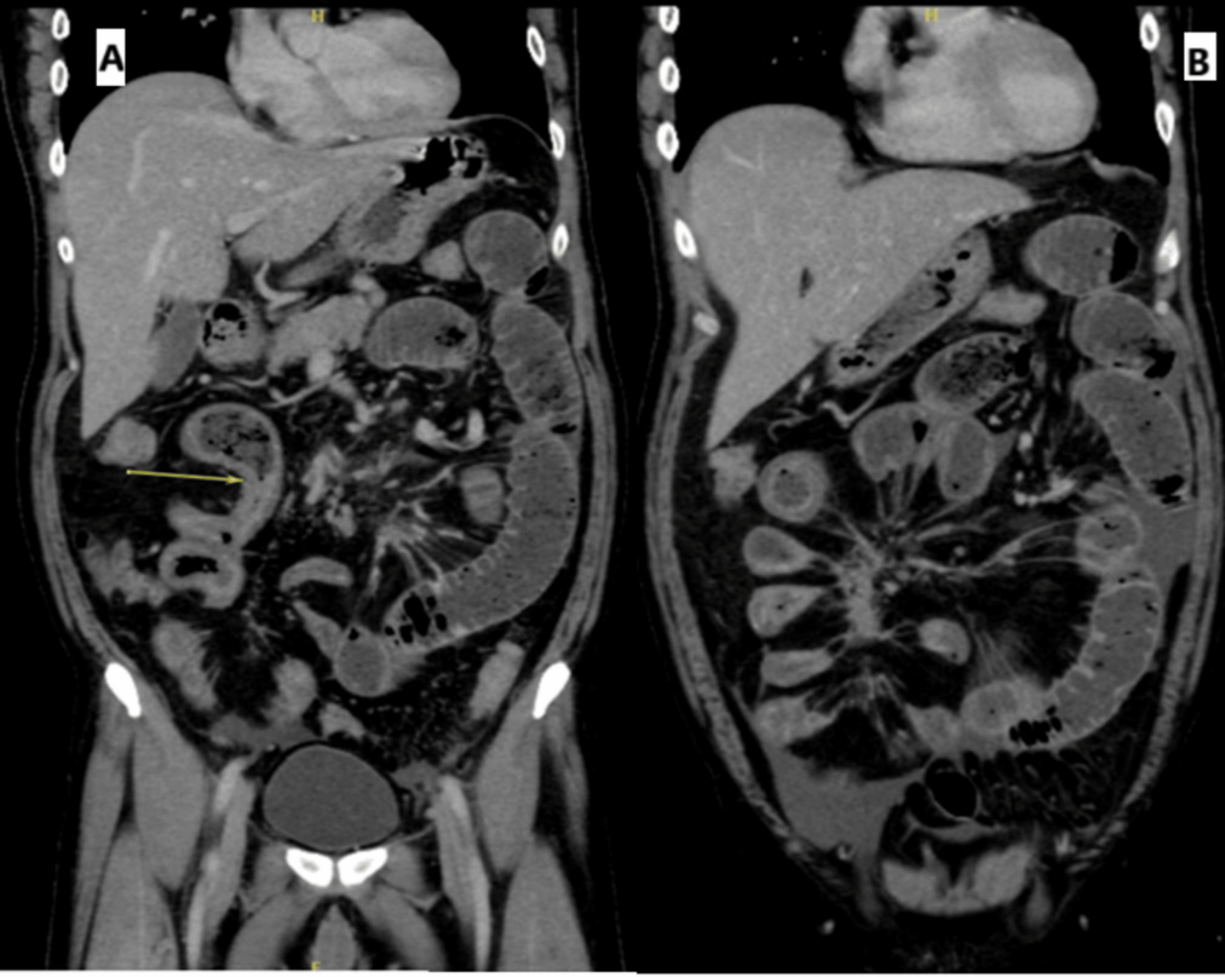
A coronal CT scan showing diffuse dilatation of the small bowel loops measuring up to 4.6 cm within the transition zone It is a long segment of distal/terminal ileal loops that surround and are affected by the desmoplastic reaction and kink from the known mesenteric mass, which shows interval progression of edematous wall thickening that could be reactive to the ongoing obstruction or the mesenteric desmoplastic reaction.
